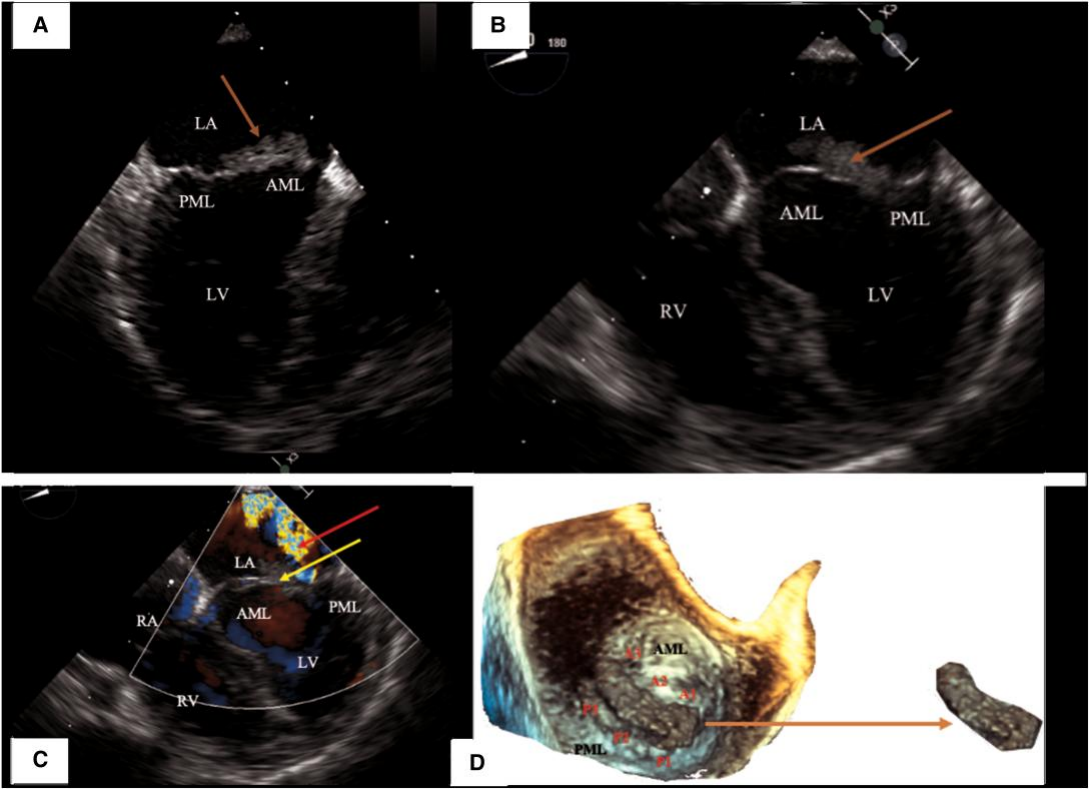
Transoesophageal echocardiography (TEE) (14 April 2023). (*A*) TEE zoom view (93°): mass measured 21× 7 mm (orange arrow). (*B*) TEE view (20°): mass measured at 21× 7 mm (orange arrow). (*C*) TEE view (20°): mitral regurgitation quantified as moderate by PISA method: SOR (Surface orifice regurgitation) 0.25 cm^2^, VOR (Volume of regurgitation) 34 mL, red arrow. Mass attached to AML (arrow). (*D*) TEE anterior view of the mitral valve—3D reconstruction (120°): mass attached to the anterior mitral leaflet (arrow). Anatomical landmarks: AML, anterior mitral leaflet (A1–A3); PML, posterior mitral leaflet (P1–P3); LA, left atrium; LV, left ventricle; RV, right ventricle; RA, right atrium.
